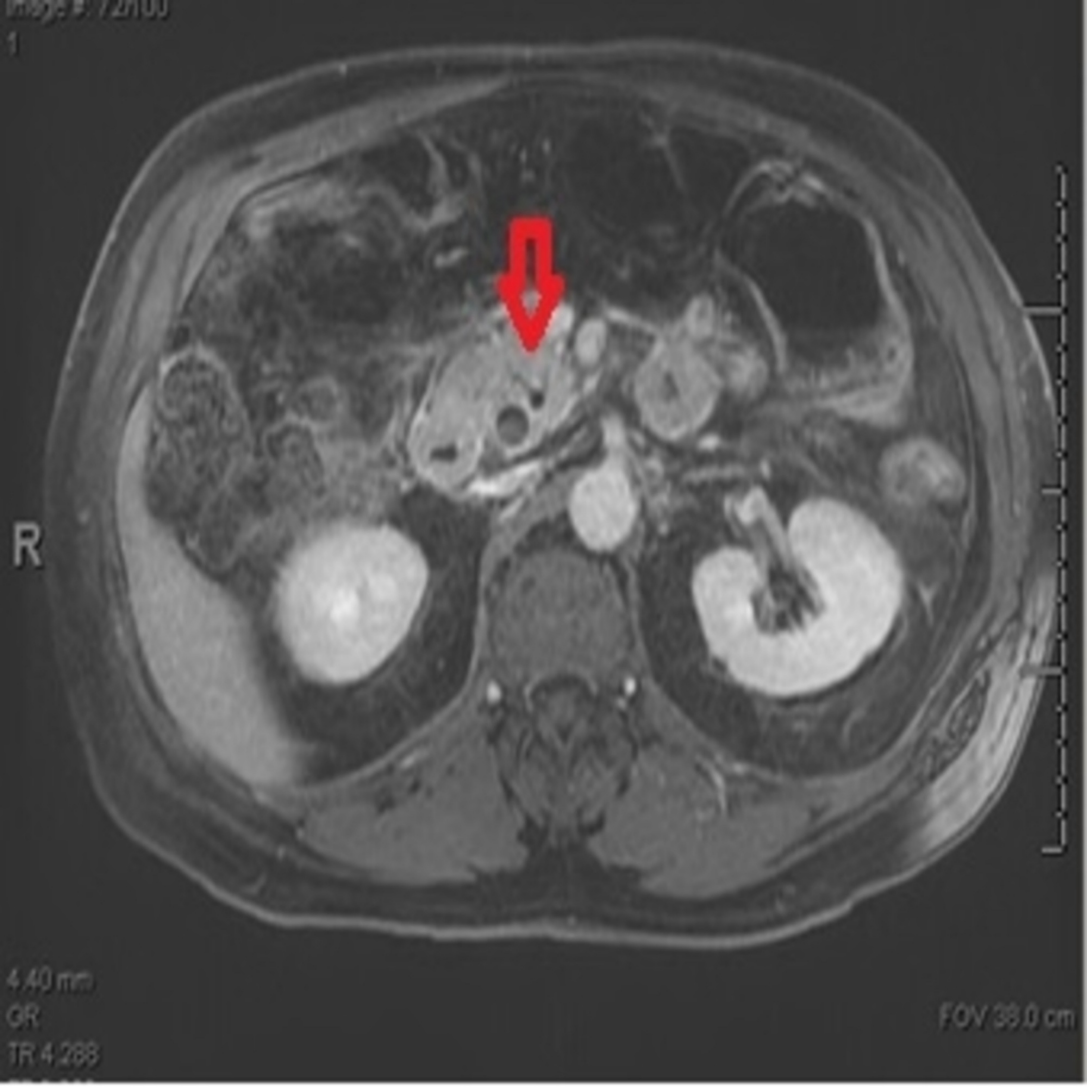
MRI abdomen showing double duct sign magnetic resonance imaging (MRI)
